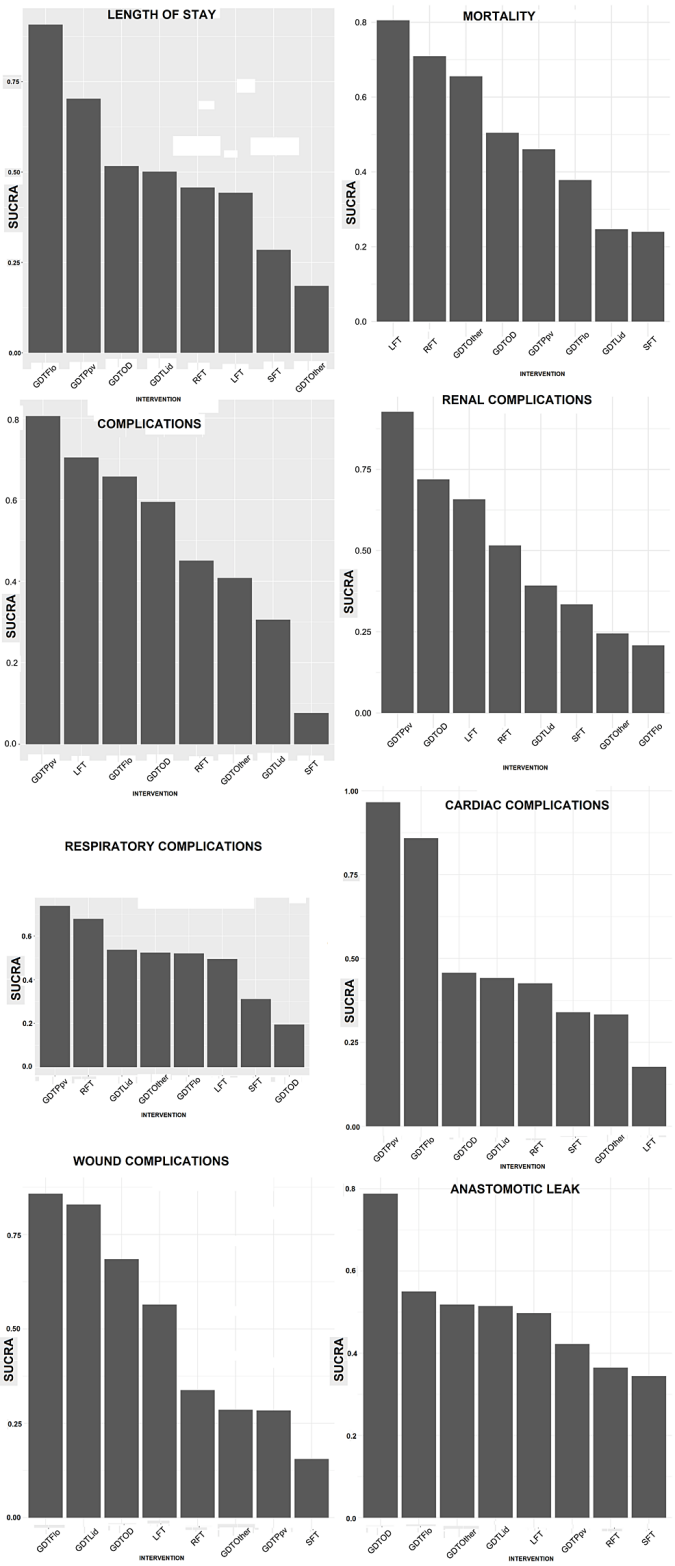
Graphs of SUCRA score (y-axis) for each intervention (x-axis) for each complication SUCRA, surface under cumulative ranking curve; goal-directed therapy, GDT; GDTOD, GDT utilising oesophageal Doppler; GDTFlo, GDT using Vigileo/FloTrac; GDTLid, GDT utilising LiDCO; GDTPpv, GDT utilising pulse pressure variation or pulse variability index; GDTOthers, GDT utilising other technology; LFT, liberal fluid therapy; SFT, standard fluid therapy; RFT, restricted fluid therapy
